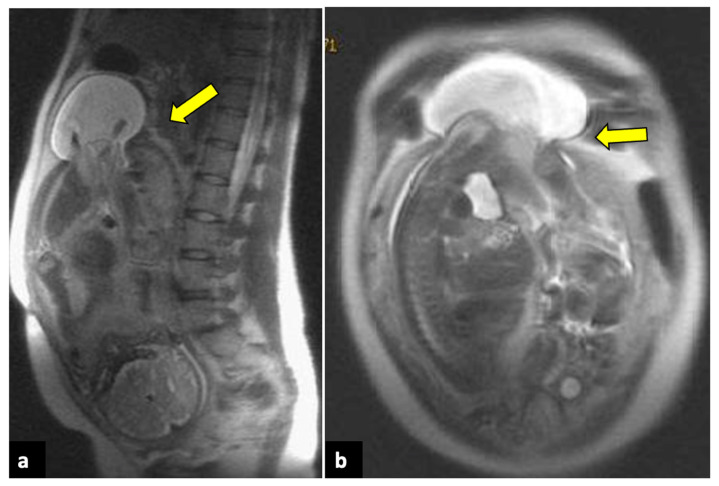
Uterine rupture in a thirty-three-year-old patient presented at the emergency department with acute pelvic pain and abnormal uterine contractions during the 37th week of pregnancy. The patient had a prior history of two previous uterine curettages. MRI T2-weighted sagittal (a) and coronal (b) images show a complete division of the uterine fundus wall with extrusion of the gestational sac (yellow arrows).
